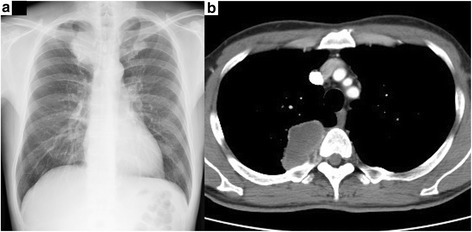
Radiologic imaging of the chest of a 48-year-old man with pleomorphic carcinoma of the lung. a Chest radiograph reveals a 6-cm shadow in the right upper lung field. b Chest computed tomography reveals a 6.5-cm tumor in the right upper lobe with invasion to the chest wall
